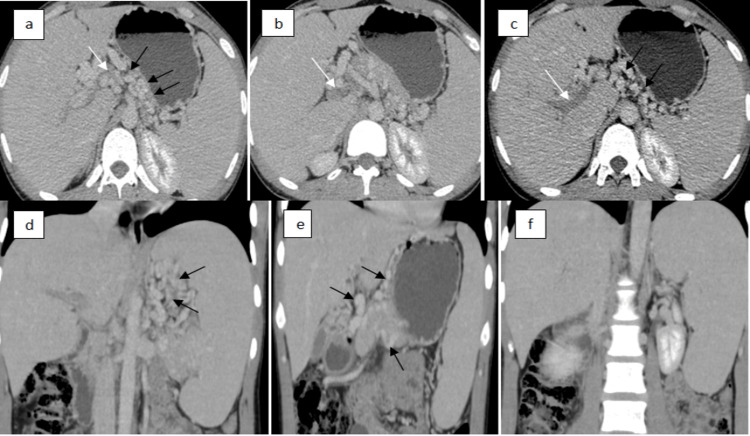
Contrast-enhanced computed tomography scan of the abdomen (a and b) Axial venous phase images showing absent enhancement of the main portal vein (white arrows), suggestive of complete thrombosis; (c) axial venous phase image showing complete thrombosis of the right branch of the portal vein (arrow), with multiple portal collaterals seen in (a) to (c) (black arrows); (d and e) coronal venous phase images depicting multiple portal collaterals around the portal vein, lesser sac, and along the lesser and greater curvature of the stomach (black arrows); (f) significant hepatosplenomegaly
